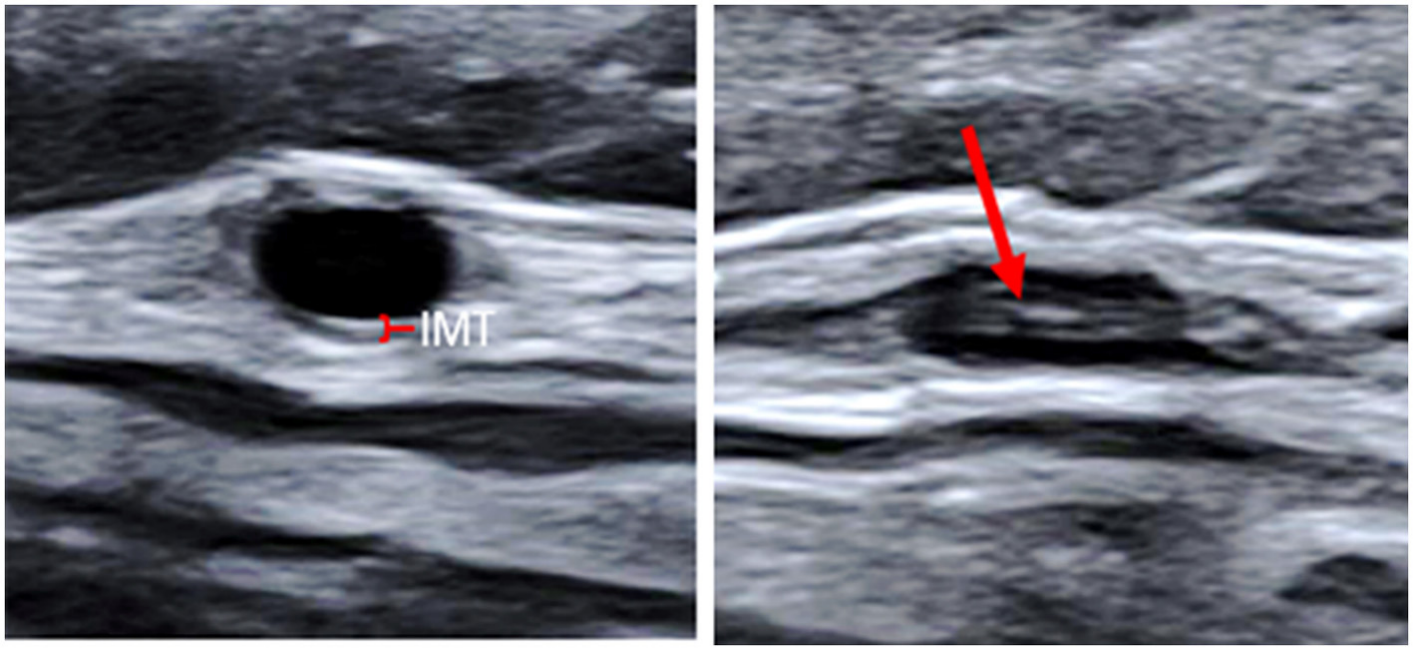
Transverse ultrasound images of the common superficial temporal artery. Non-compressed to the left (the red mark indicates the normal thin IMT), and compressed to the right. The high-echogenic part (red arrow) in the middle of the compressed vessel is the intima from the near and far wall pressed together. IMT, Intima-media thickness.
